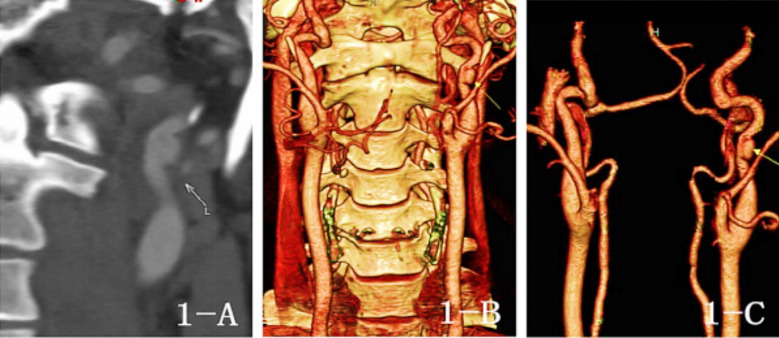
Preoperative CTA images Fig.1-A: (MIP), Fig.1- B, 1-C: (Reconstruction Image) revealed left extracranial carotid artery aneurysm, Size: 5*8mm
